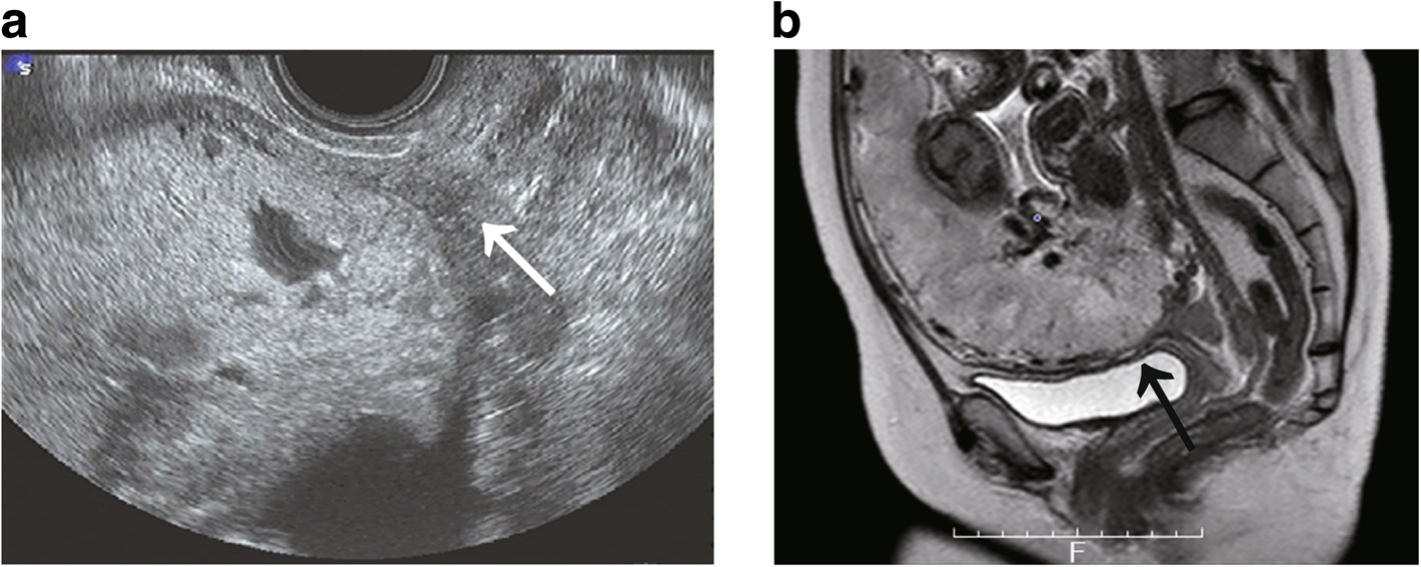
Images for assessment of placenta accreta spectrum. a Transvaginal ultrasonography shows total placenta previa with one lacuna. b Magnetic resonance imaging (MRI) at gestational week 31 revealed total placenta previa, and the placenta was located mainly on the anterior side. Although intraplacental T2 dark band, uterine bulging, and heterogeneous placenta were not observed, we found myometrial thinning of the anterior wall and loss of myometrium between the placenta and bladder wall. The black arrow indicates loss of uterine myometrium between the placenta and bladder wall. Based on these findings, we suspected placenta accreta spectrum. No abnormal finding was observed in the fetus
